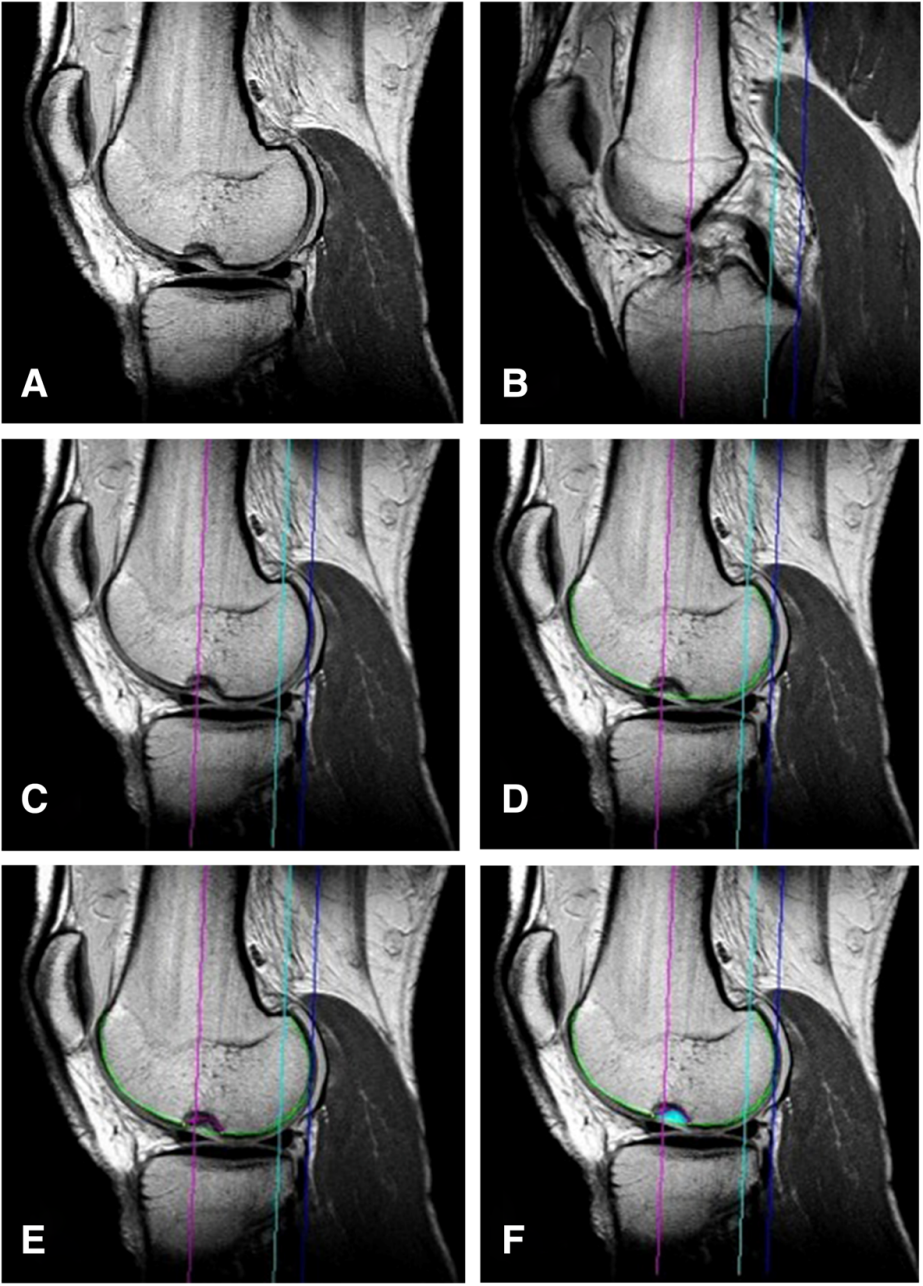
Segmentation of the MRI. (a) sagittal MRI with lateral femoral notch. Alignment of the axis: (b) line parallel to the axis of the femoral shaft running through the deepest point of the intercondylar notch (pink line), (c) most posterior aspect of the lateral femoral condyle (dark blue line), “weight bearing area” (light blue line), both parallel to the axis of the femoral shaft. (d)Segmentation of the defect: the subchondral bone area (green line), (e) marking of the defect (curved pink line), (f) area of the defect (light blue area)
